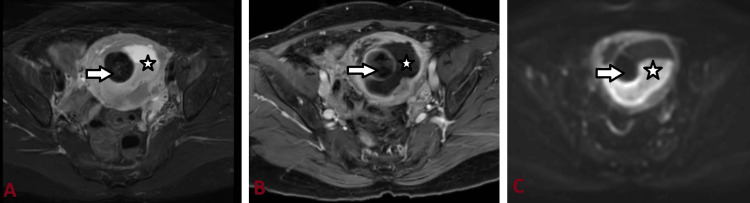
MRI abdomen and pelvis T2-FS (A), T1-C+ (B), and DWI axial sections showing heterogeneously hyperintense endometrial collection (star) and restricted diffusion in DWI (C) suggestive of pyometra. A submucosal fibroid (arrow) can also be seen adjacent to the endometrial collection. FS: Fat saturated; C+: Contrast; DWI: Diffusion-weighted imaging
